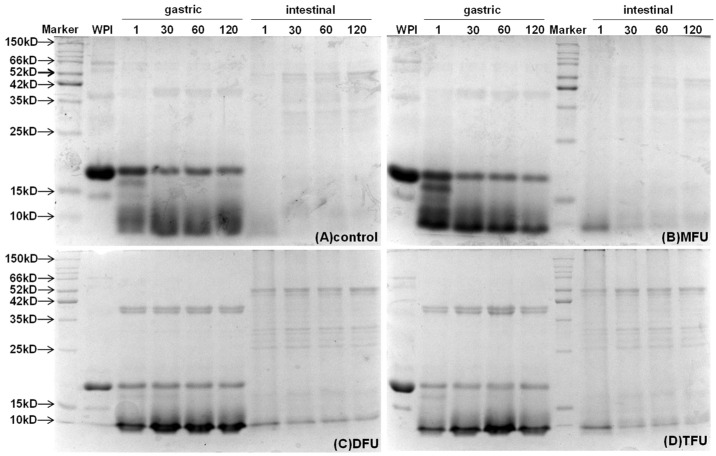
SDS-PAGE of ultrasound pretreated whey protein gels during gastric and intestinal digestion at different time points (1, 30, 60, 120 min) (control: without ultrasound, MFU: mono-frequency ultrasound, DFU: dual-frequency ultrasound, TFU: tri-frequency ultrasound).
